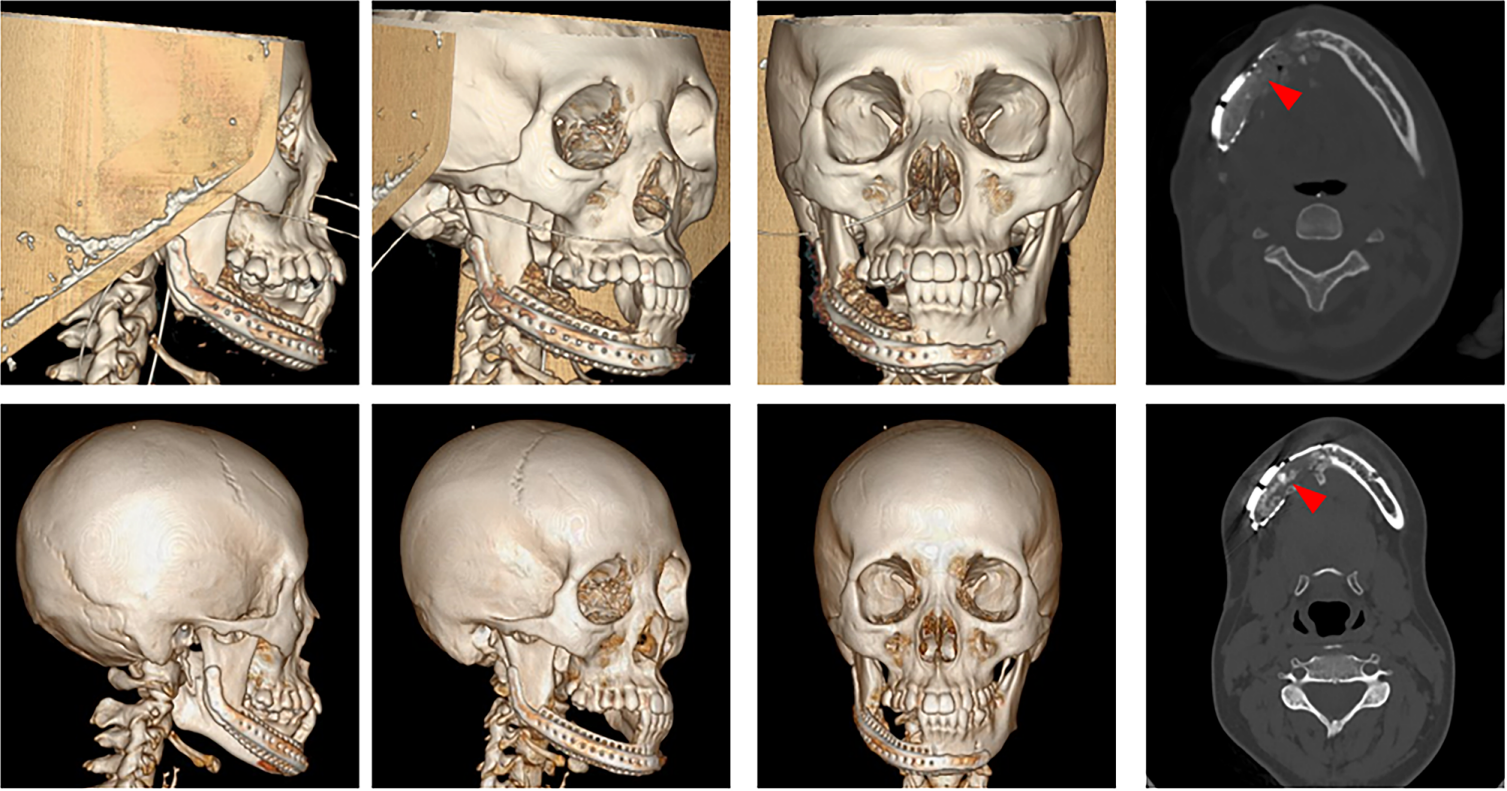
The CT images at seven days post-operation (upper) and six months post-operation (lower) Additional CT images taken at the same horizontal position were also displayed, with red arrows indicating the cancellous bone of the ilium
